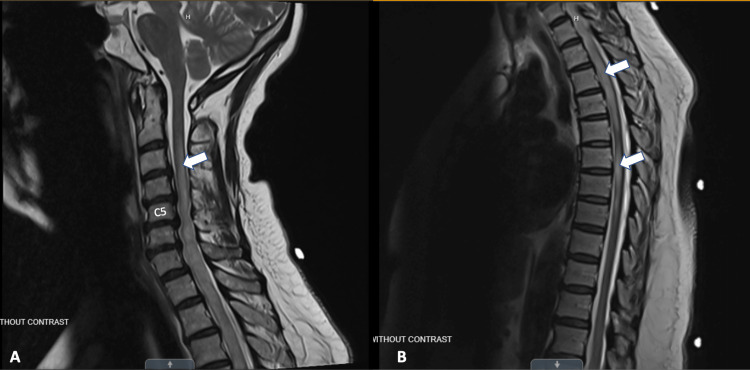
Cervical and thoracic T2 MRI. (A) T2 cervical spine MRI-transverse myelitis in the cervical region with the extension of cord signal abnormality (white arrow) with mass effect on the cervical cord at C5-C7 (C5 labeled on image) due to worsening cord swelling. (B) T2 thoracic spine MRI-areas of T2 bright signal (white arrows), cord expansion and cord enhancement in the thoracic region extending down to the T8 level. MRI: magnetic resonance imaging.
